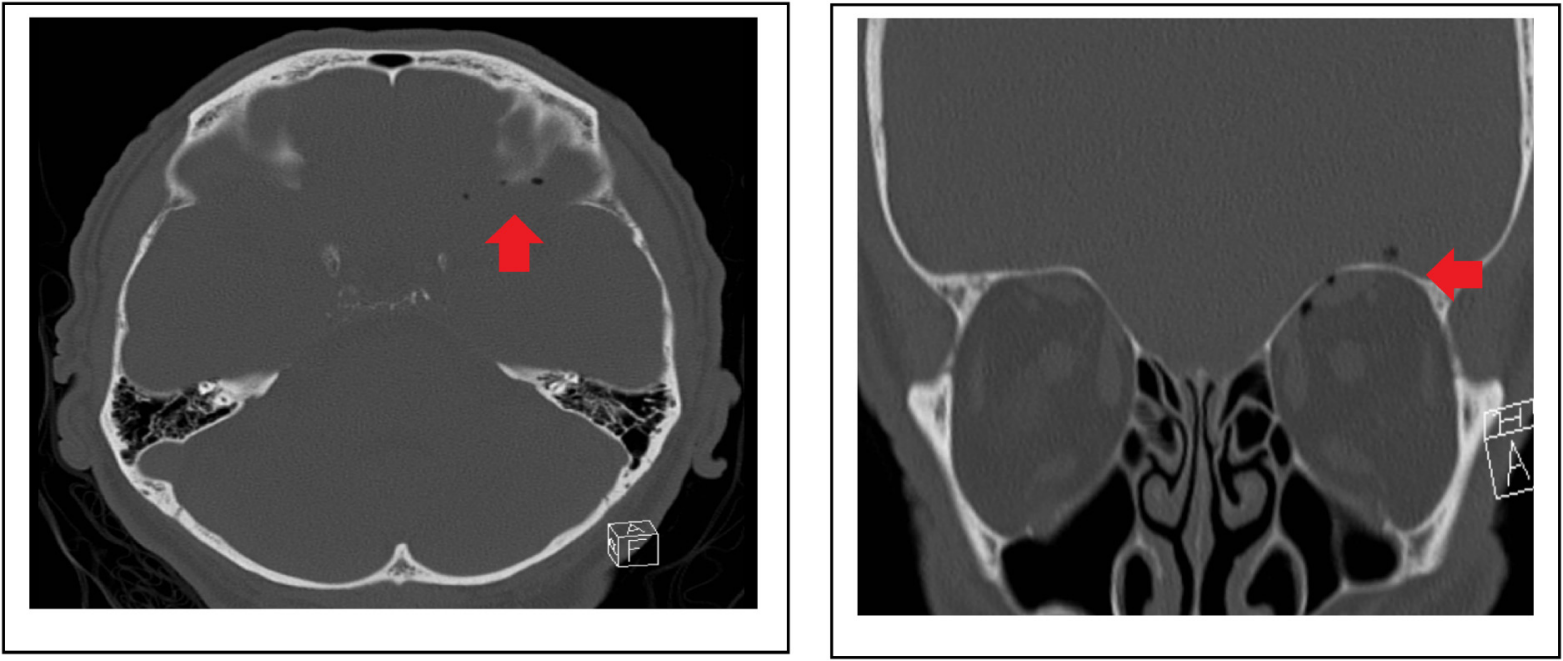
cranial CT showing intra-cranial air with suspicion of a small fissure at the upper orbita and a suspicion of blood in the left fronto-basal sulcus.
